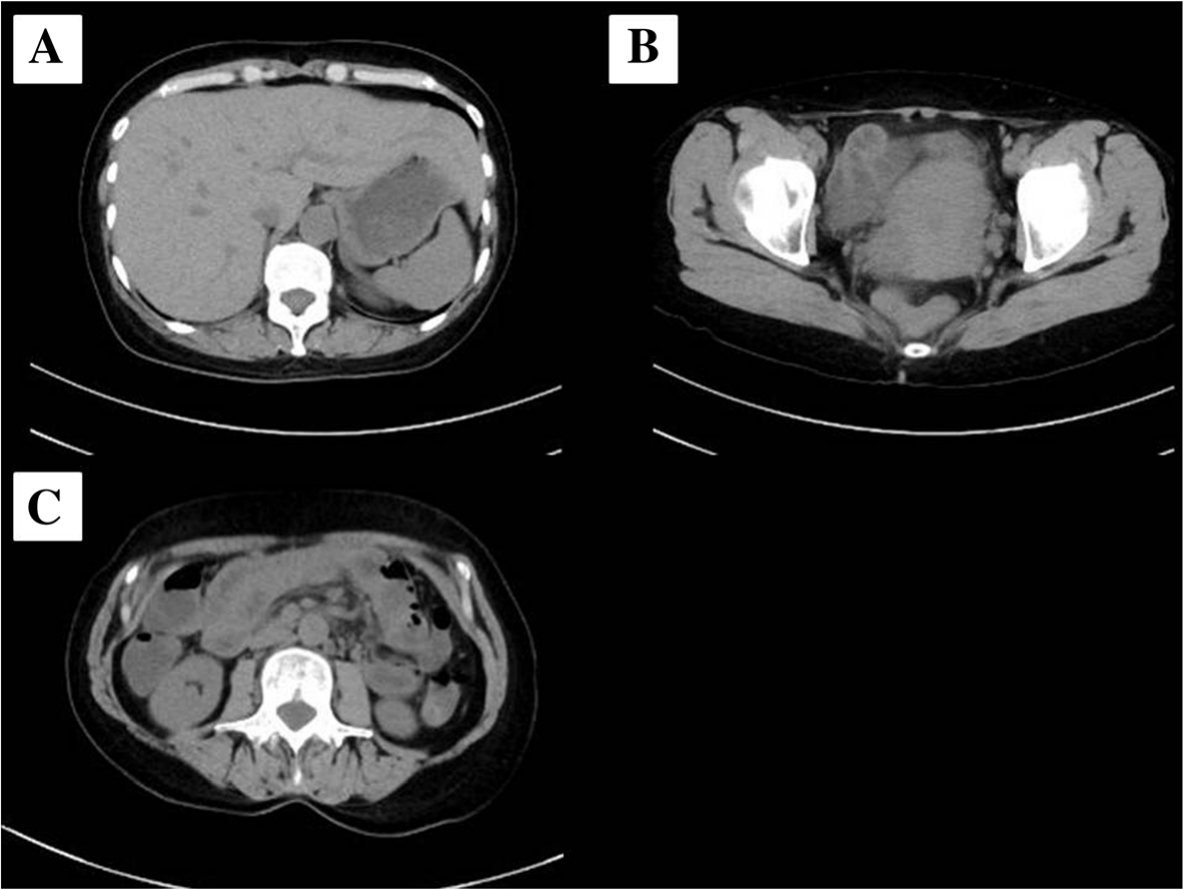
Abdominal computed tomography examination on admission. Abdominal computed tomography examination on admission showed no ascites (a, b) and a thickened wall throughout the entire small intestine (c)
